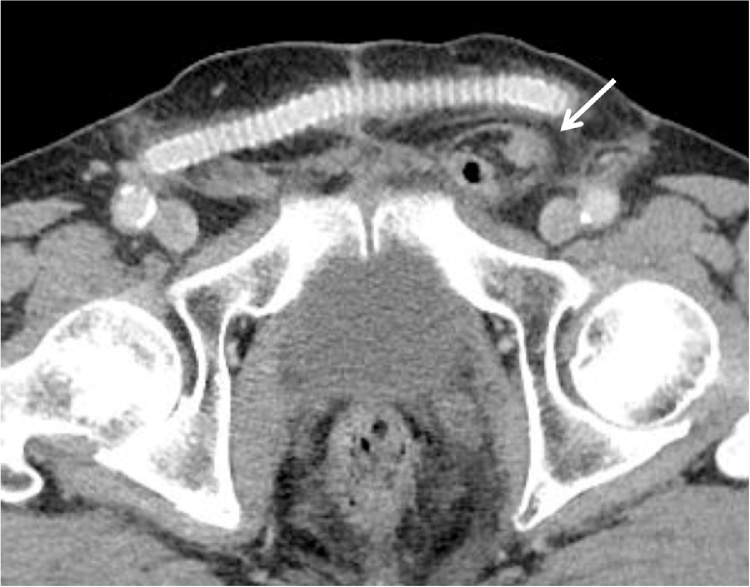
Case 2. Computed tomography revealed a left inguinal hernia (arrow) and femoro-femoral arterial bypass graft in the subcutaneous plane.
